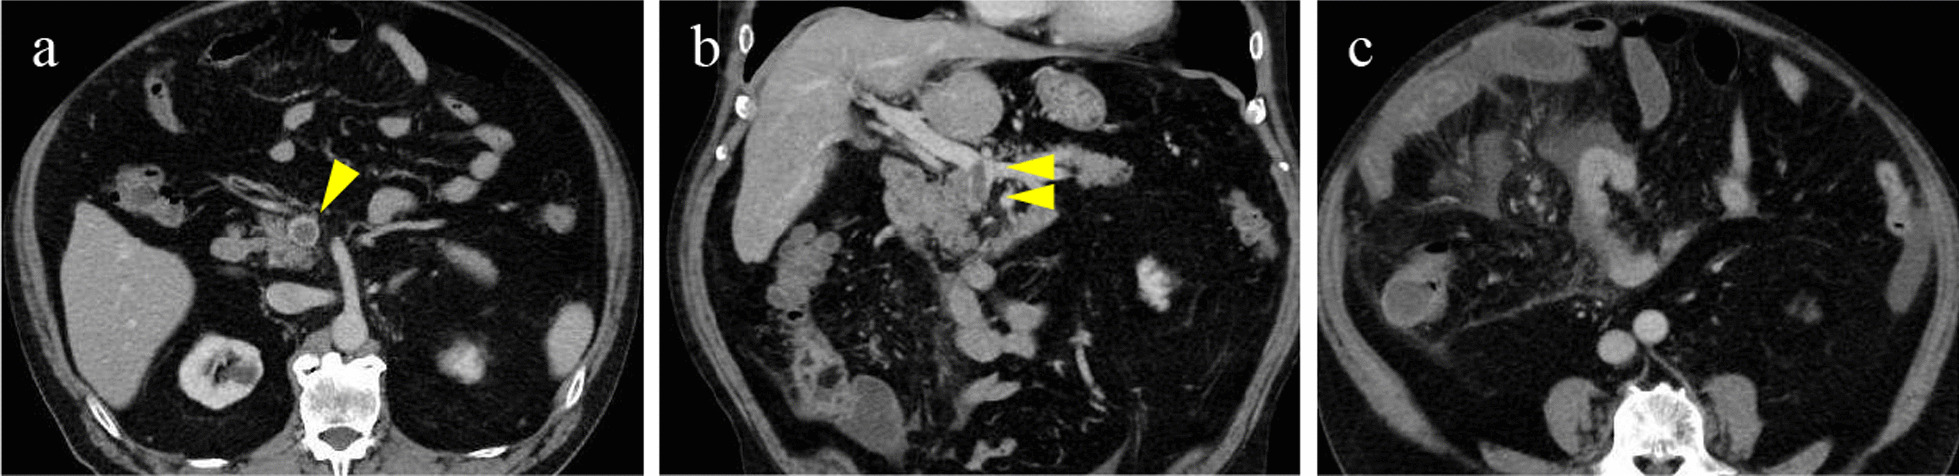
Abdominal computed tomography on admission. Yellow arrows: Thrombosis in the superior mesenteric vein. Axial image (a). Coronal image (b). Increased adipose tissue density in the mesentery of the small intestine, focal edema of the small intestine, and ascites around the small intestine (c)
